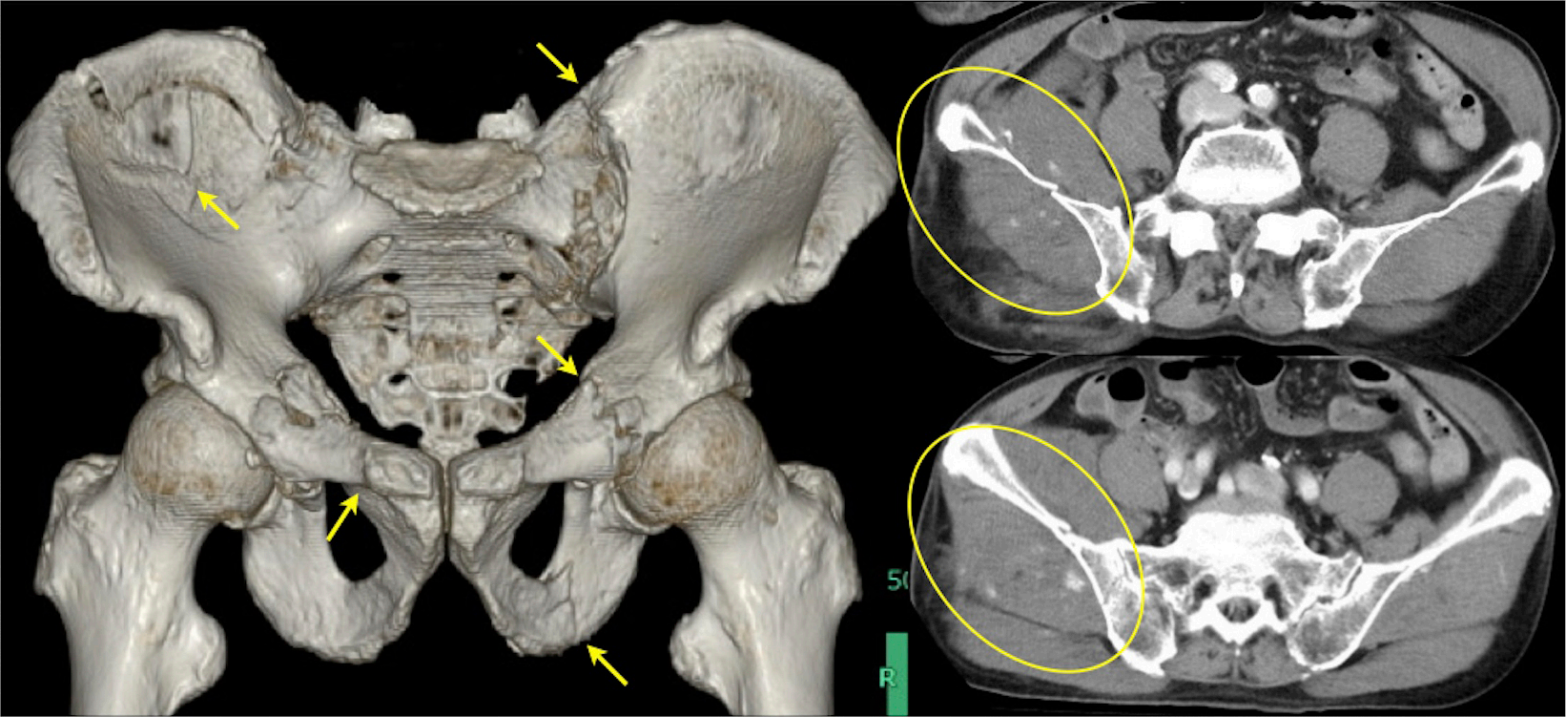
Computed tomography (CT) of the pelvis revealed a pelvic fracture classified as LC-III according to the Young-Burgess classification (arrow). Contrast-enhanced CT also revealed contrast medium extravasation in the pelvic muscles (circle).
